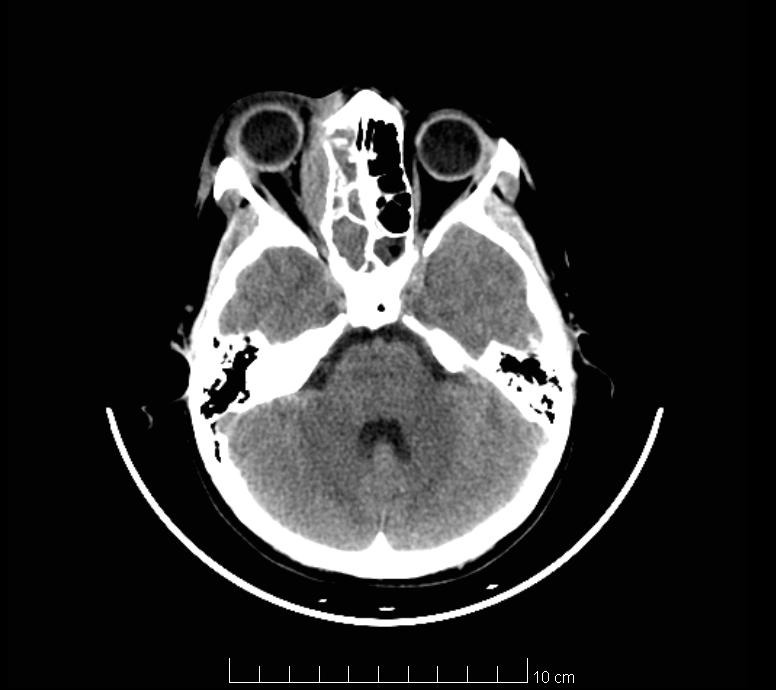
Preoperative Head CT-Scan (blue arrow – orbital cellulitis and osteitis, red arrow - sinusitis)
